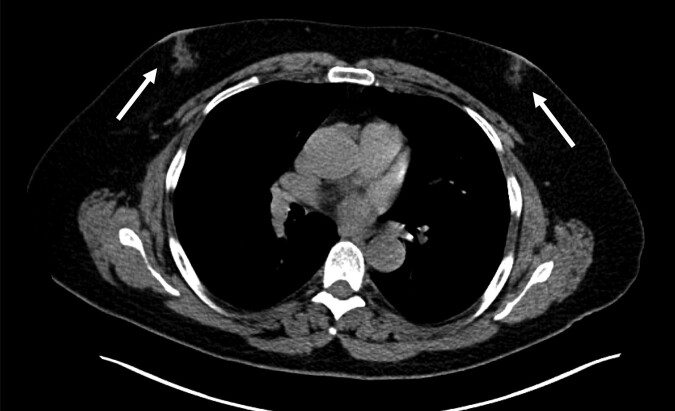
Unenhanced CT through the chest demonstrates bilateral gynecomastia (arrows).
